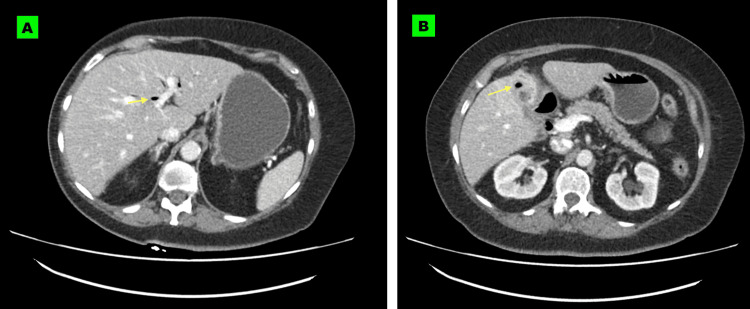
Axial CT slices of the upper abdomen show air in the biliary tree (pneumobilia) (A) and air in the gallbladder fossa (B) CT: Computed tomography
